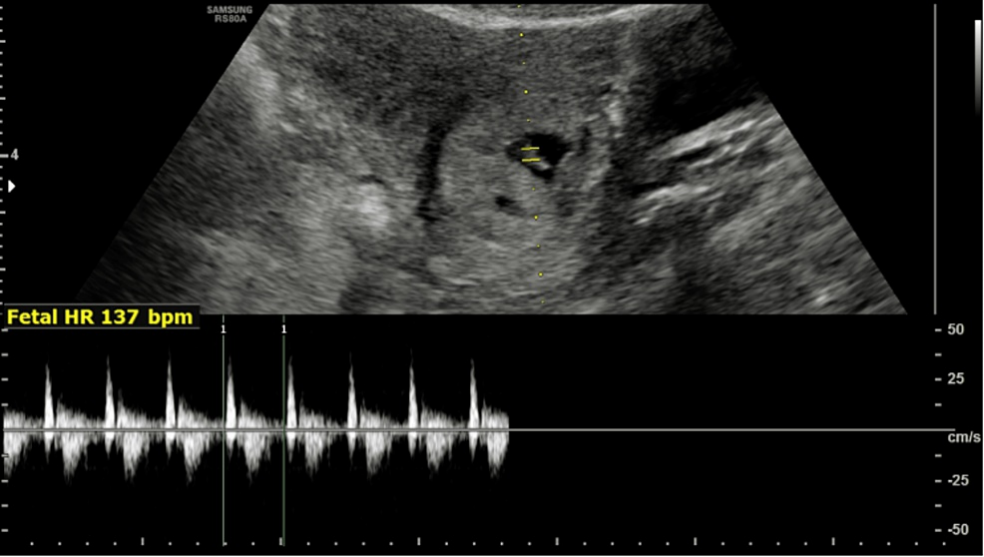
Transabdominal ultrasonography with spectral Doppler showing cardiac pulsations of 137 bpm. bpm: beats per minute, HR: heart rate
